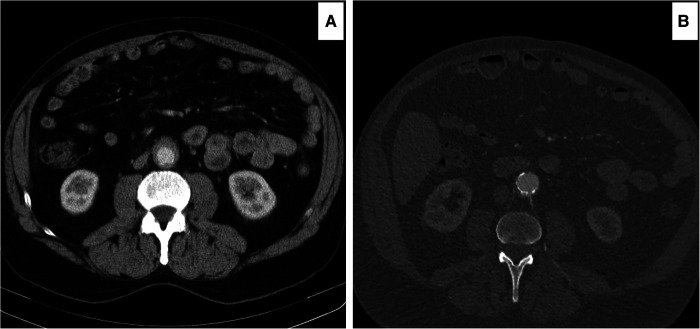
Computed tomography angiography (CTA) images showing decrement of the peri-aortic soft tissue enhancements following medical management. (A) Initial 4 mm peri-aortic soft tissue enhancement. (B) Two years post medical management, the peri-aortic soft tissue enhancement was reduced to 1 mm.
